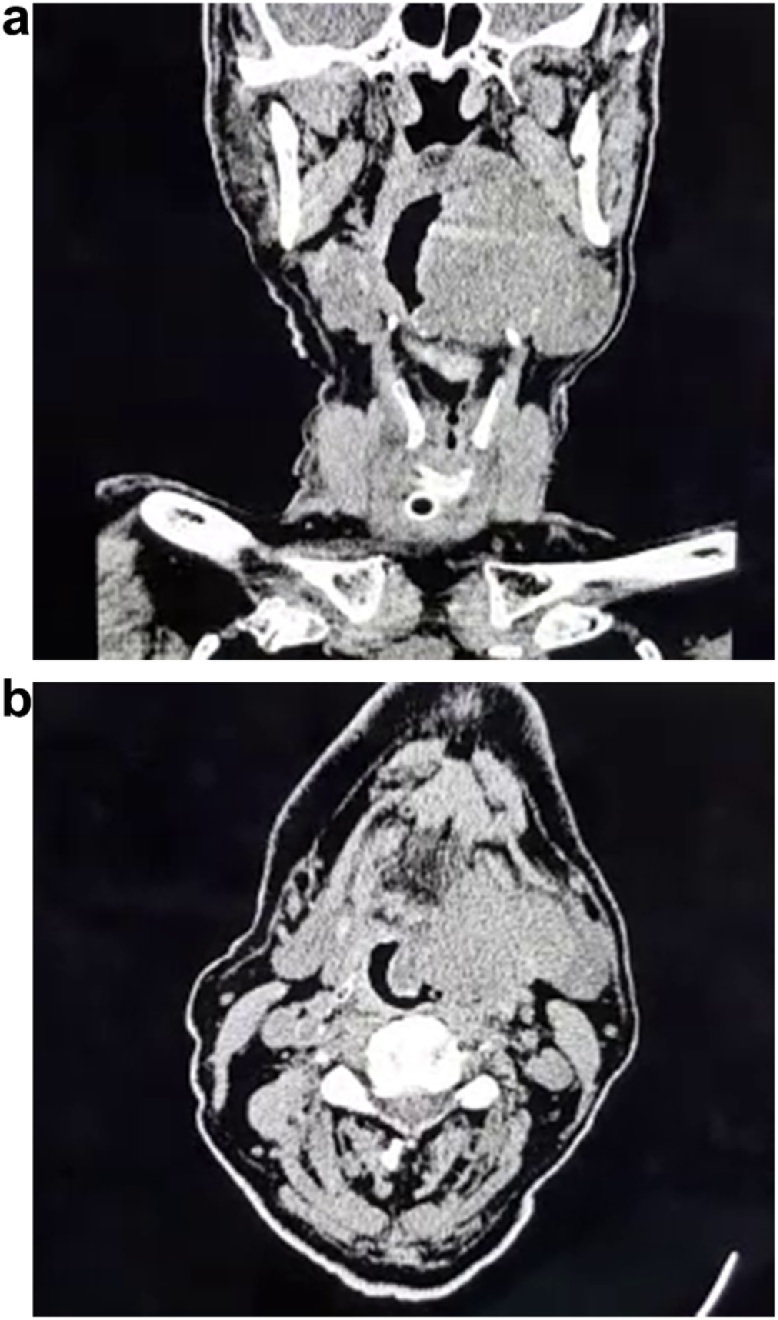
(a) CT scan of the face and Neck (Coronal view); (b): CT scan of the face and Neck (Axial view)- showing 7.4 cm x 6.9 cm x 4.6 cm solid mass at the base of the tongue as denoted by the white arrows.
